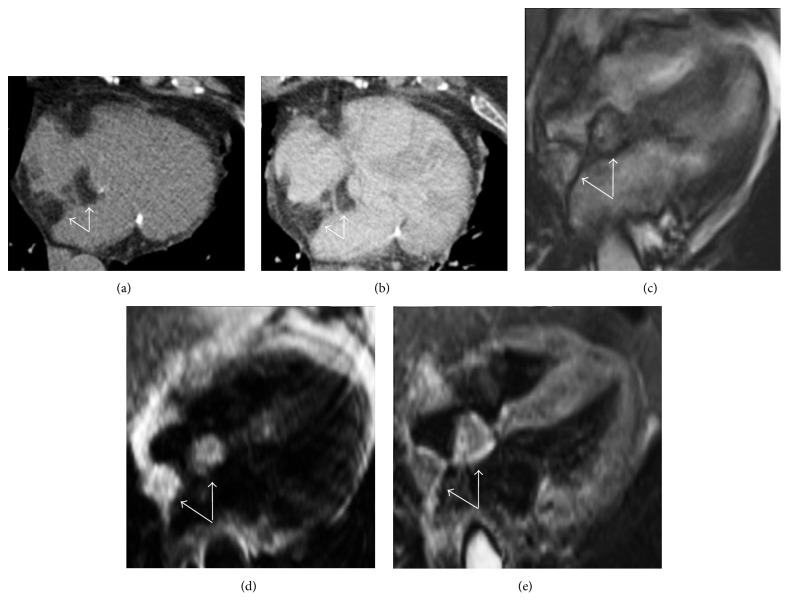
Lipomatous hypertrophy of the interatrial septum as incidental finding in an asymptomatic 72-year-old female. CT imaging of the heart in precontrast scan (a) and after contrast medium administration (b) in axial views demonstrates a bilobular dumbbell shape hypoattenuating lesion with lobular morphology located within the interatrial septum that appears thickened (arrows). CMR examination requested for a further evaluation confirms the presence of a bilobular mass with corresponding hyperintensity in four-chamber cine-SSFP sequence surrounded by the typical black boundary artefact ((c) arrows) as in T1-weighted black blood image ((d) arrows), with low signal on black blood T2-weighted sequence with fat suppression (arrows).
